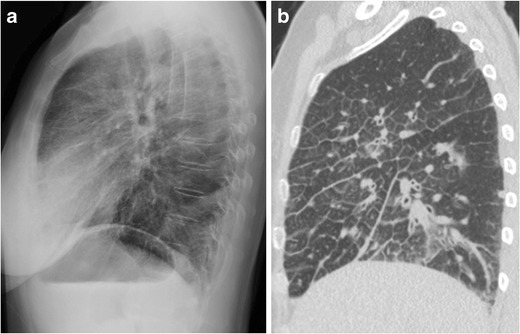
62-year-old woman with metastatic lung adenocarcinoma and lymphangitic carcinomatosis. Interlobular septal thickening is clearly seen in the retrosternal clear space on the lateral radiograph (a). A corresponding sagittal CT slice (b) is also shown
